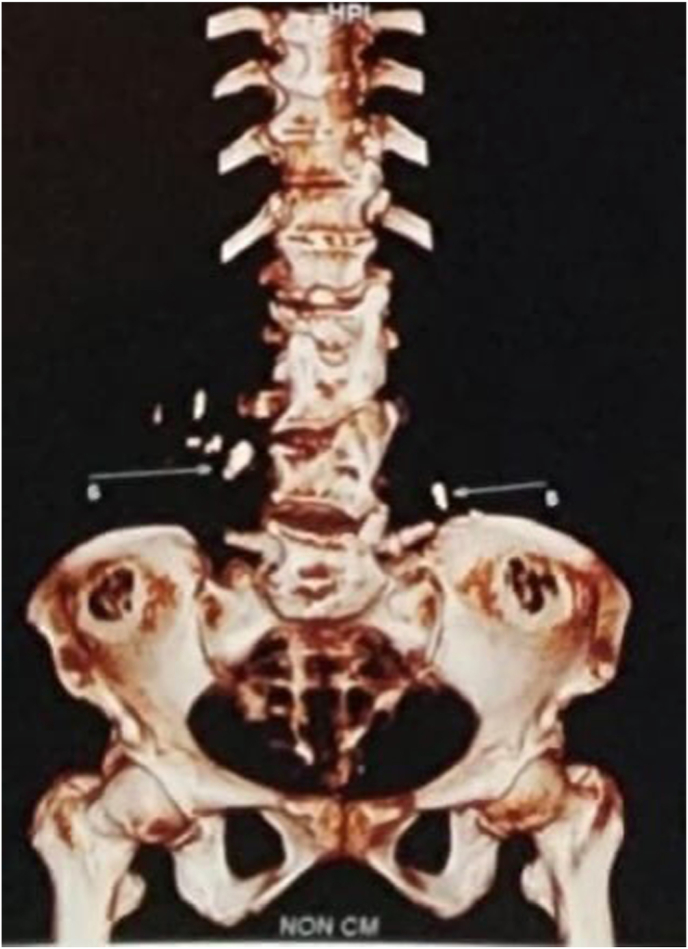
CT scan reconstruction confirming multiple kidney stones and bilateral ureteral stones.
